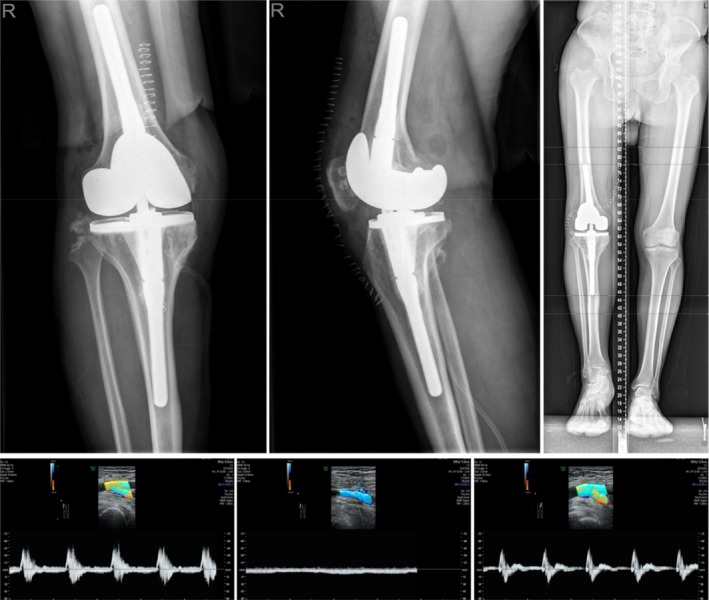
(A) X‐rays of post right TKR. Status post right knee total replacement shows acceptable implant alignment, size, and position. There are no fractures, translations, or dislocations. Moderate joint effusion is present. Multiple skin staples, soft tissue edema, and air foci are consistent with early postoperative changes. (B) Doppler ultrasound of the arteries and veins in both lower limbs after TKR reveals a normal inner diameter of the arteries in both the right and left lower limbs, with thickened and uneven intima. Multiple small, strong light spots can be seen on both arterial walls, accompanied by comet tails. There is no stenosis in the arterial lumen, and Doppler blood flow filling in the arteries is good.
